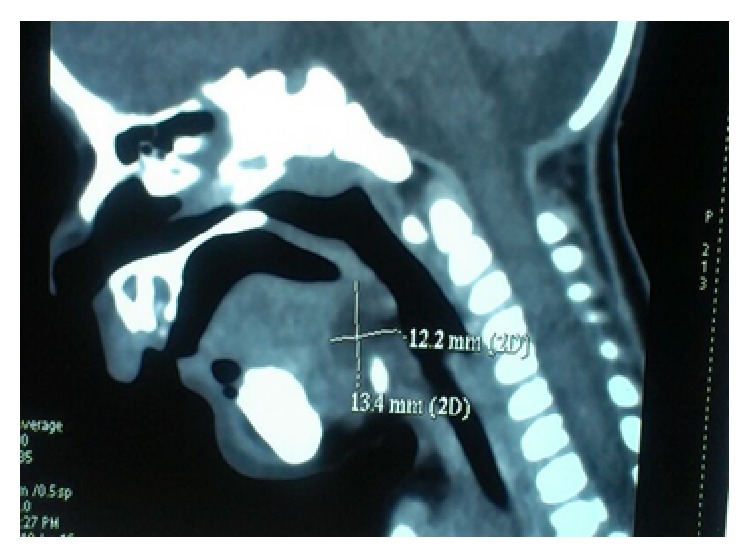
Contrast enhanced computed tomographic scan showing nonenhancing fluid filled lesion located at base of tongue of 12.2 × 13.4 mm.
